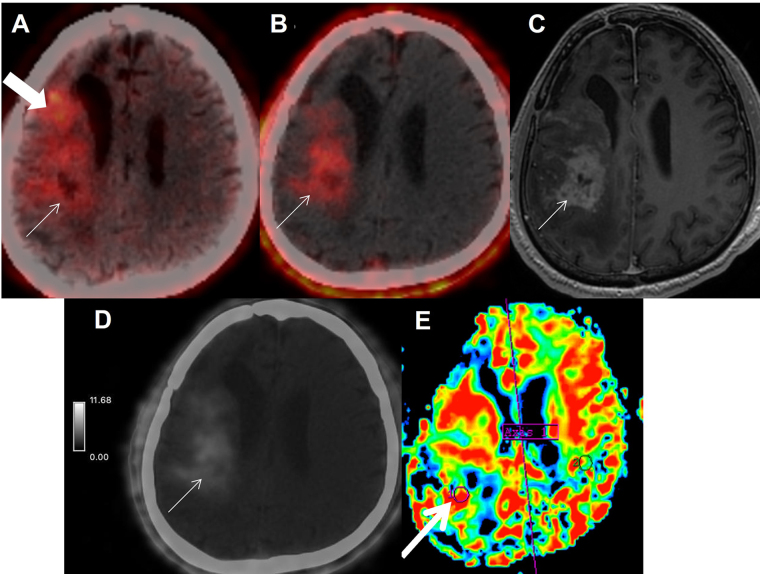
Patient 2 is a 66-year-old male, case of right temporal WHO Grade IV GBM, post surgery, radiation therapy with concurrent and adjuvant temozolamide (completed in January 2019), presented in June 2021 with new-onset seizures. MRI and FET PET was advised. (A) Axial FET PET showed tracer uptake in the right fronto-temporal and insular region (arrow), extending to anterior periventricular region (block-arrow). T-W ratio was 4.07 suggestive of disease recurrence. (B) Axial FAPI PET/CT image showed tracer uptake in the right fronto-temporal and insular region (arrow), with SUV_max_ of 2.8. It corresponded to the enhancing lesion on axial post contrast T-1 weighted image (C, arrow), which showed focal hyperperfusion (E-arrow) gray scale image of axial FAPI PET with intensity map (D, arrow) shows high-grade uptake at the site of MR and FET depicted recurrence. FET uptake was also seen in the residual disease component in the anterior periventricular region (block-arrow), which was not seen on FAPI PET/CT. MRI: magnetic resonance imaging; FET: fluoro-ethyl tyrosine; GBM: glioblastoma
